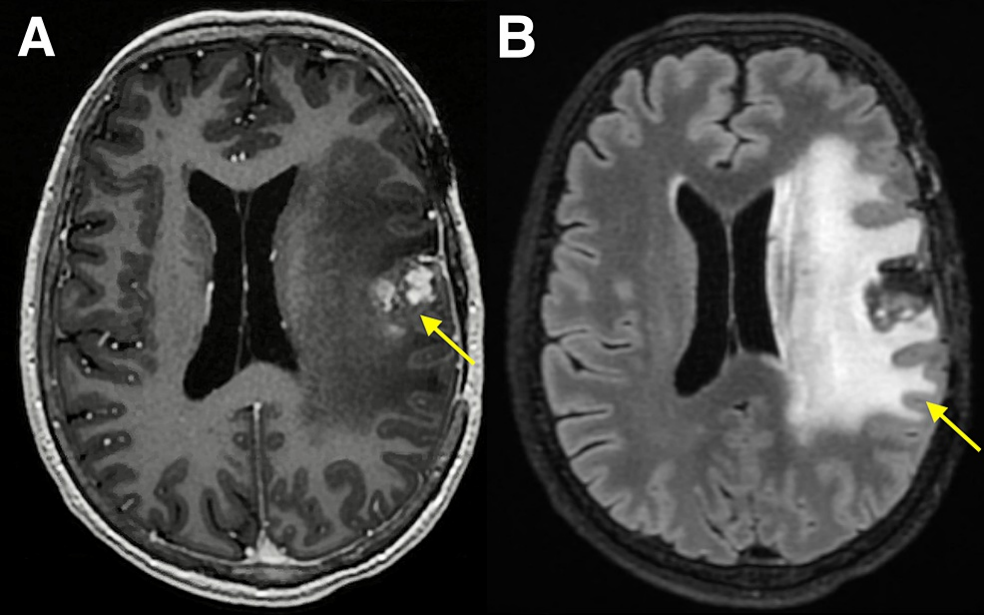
MRI 87 months after Gamma Knife stereotactic radiosurgery, 55 months after resection. Axial contrast-enhanced T1-weighted (A) and T2-weighted FLAIR brain MRI (B) demonstrating interval increase in size of the left frontal lesion, now measuring 2.6 cm, with worsening surrounding vasogenic edema and new rightward midline shift measuring 5 mm. FLAIR: Fluid-attenuated inversion recovery
